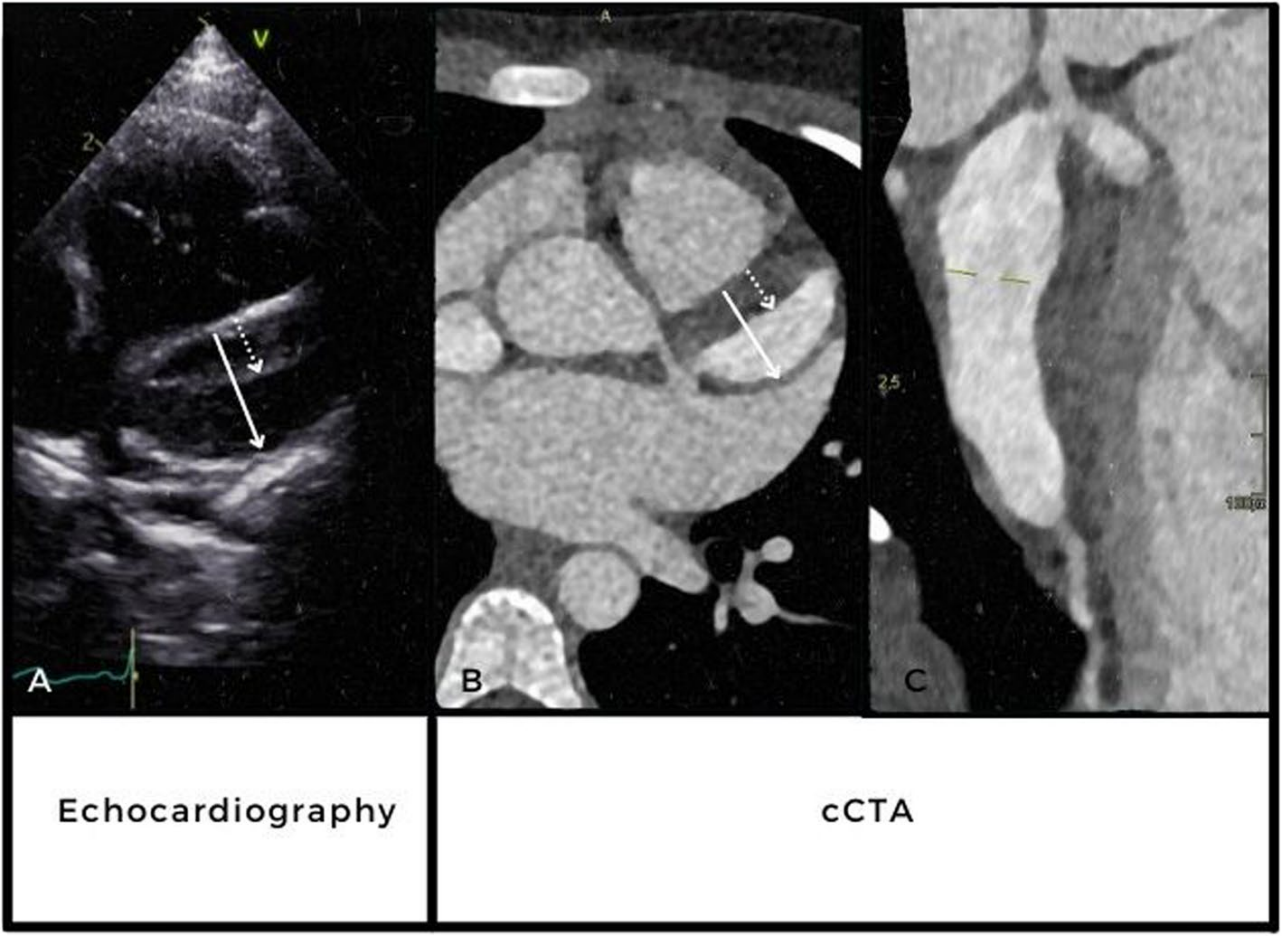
Giant CAA (arrow) in LAD with thrombus (dotted arrow) depicted by echocardiography (A) and cCTA (B + C). C A reconstruction of the full length of the left main coronary artery. Echocardiography (A) and cCTA (B) showing thrombus (dotted arrow) in giant CAA with a luminal diameter of 13 mm, equivalent to a *Z* score of 34 (arrow) in LAD
